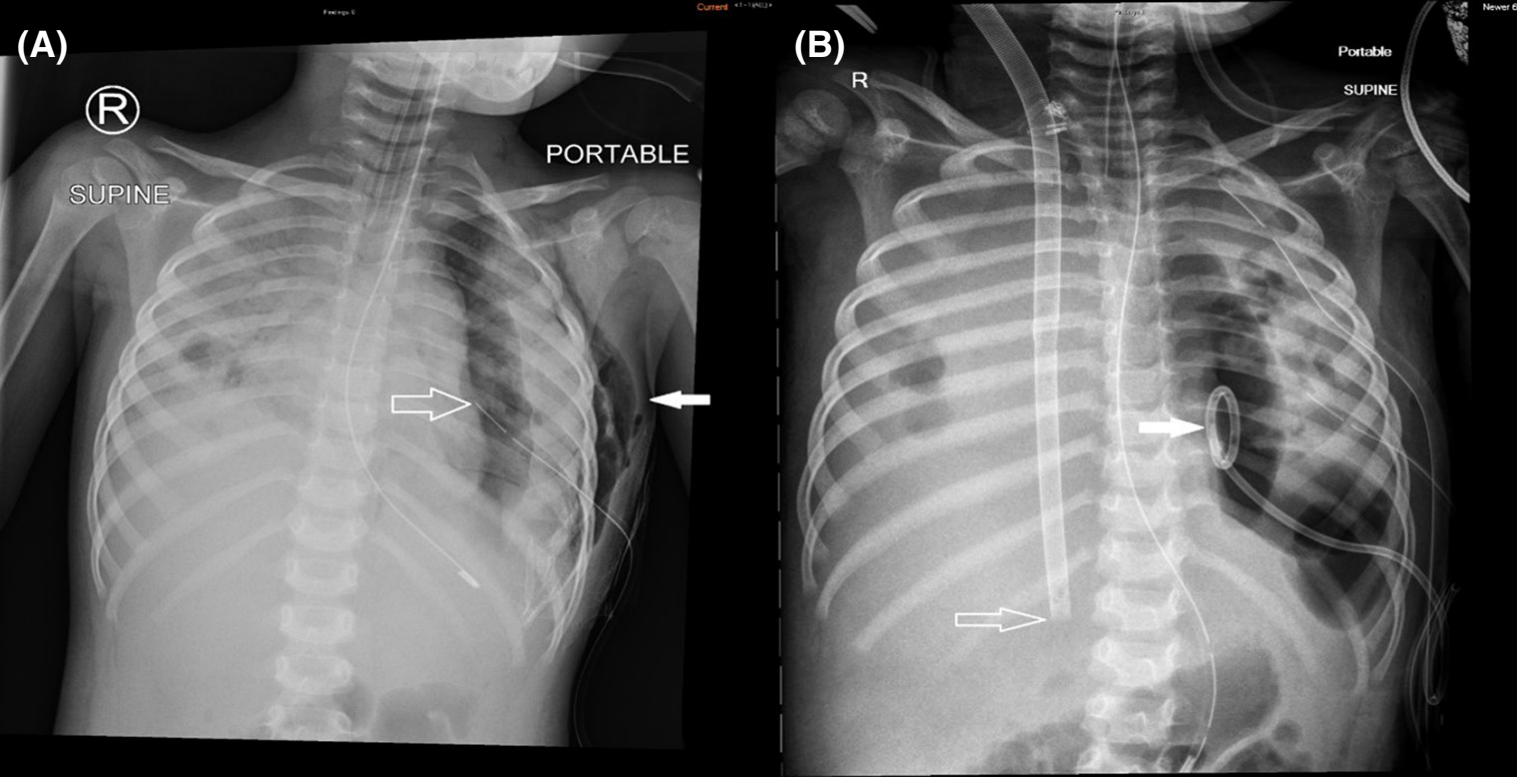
(A) Chest radiograph 4 days prior to initiation of extracorporeal membrane oxygenation (ECMO) showing bilateral consolidation, a left pneumothorax with chest drain in‐situ (open arrow) and left‐sided subcutaneous emphysema (solid arrow). (B) Chest radiograph taken on Day 6 of ECMO showing bilateral consolidation, ECMO cannula (open arrow), a coop‐loop chest drain within the left pneumothorax (solid arrow) and 2 left chest drains
